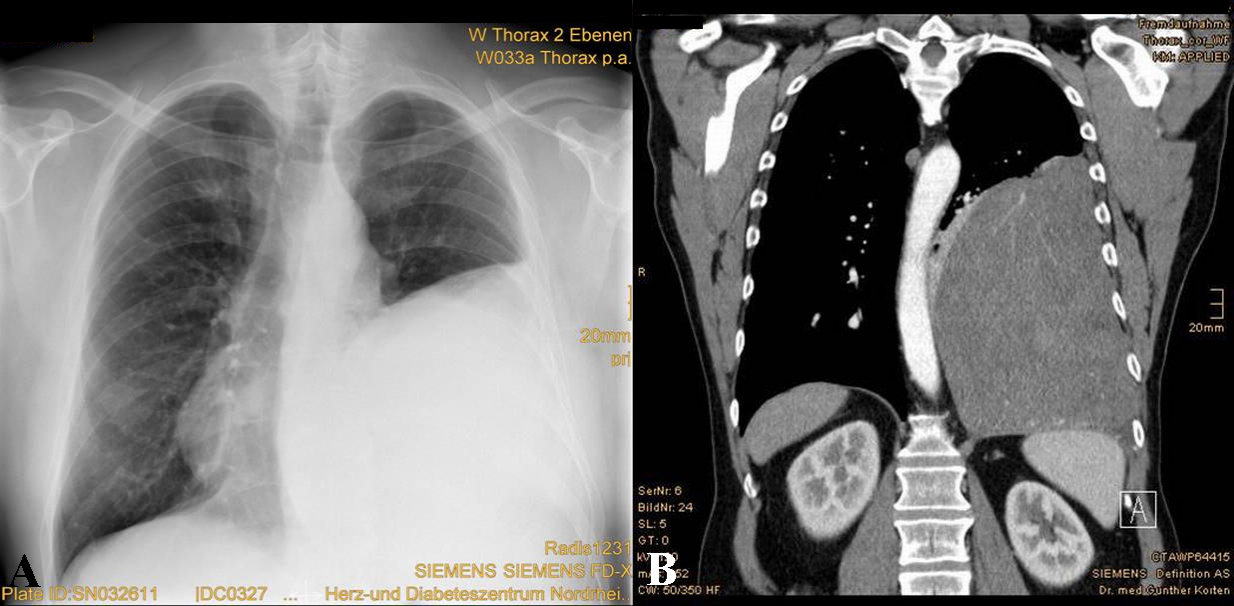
Chest radiography and CT scan images. (A) Initial chest radiography revealed a large well-circumscribed mass in the left thorax. (B) Initial contrast-enhanced computed tomography showed a huge homogeneous, sharply defined mass compressing the aorta.
